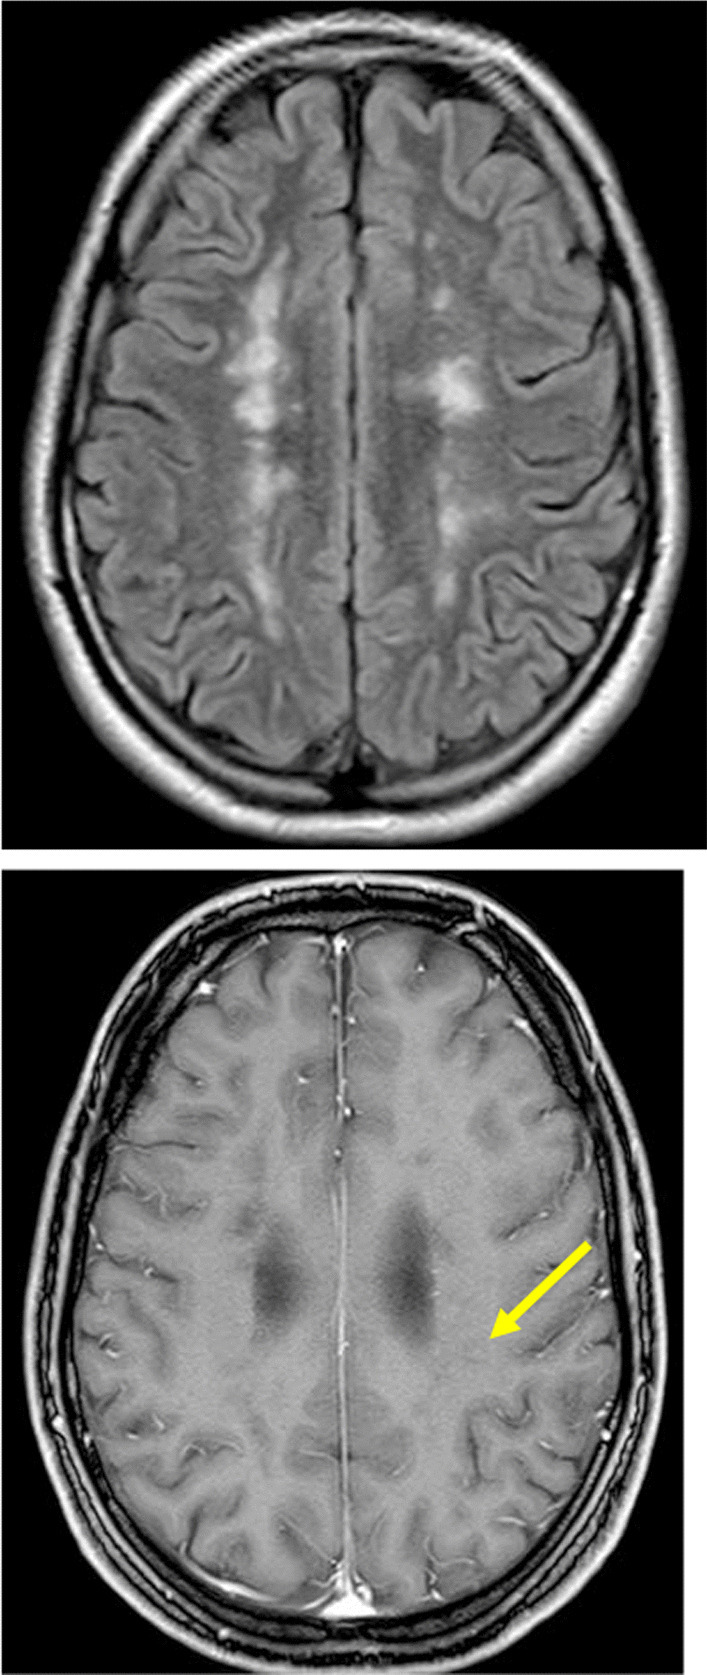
Follow-up 4 weeks after initial presentation: The FLAIR sequence depicts a reduction in size of the white matter lesions. Contrast enhancement is markedly reduced with residual patchy enhancement in the area of multifocal leukoencephalopathy (Arrow)
